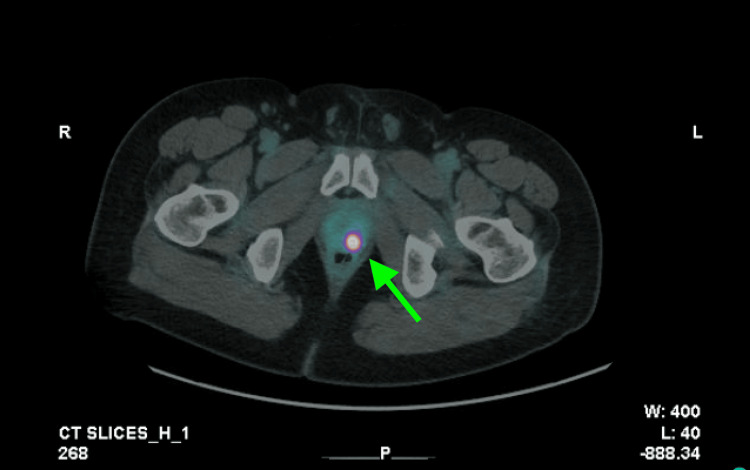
PSMA PET/CT Demonstrating Focal Uptake in the Left Posterior Prostate Axial fused gallium-68 (68Ga) prostate-specific membrane antigen (PSMA) positron emission tomography/computed tomography (PET/CT) image demonstrating focal radiotracer uptake in the left posterior prostate (arrow), corresponding to a suspicious lesion concerning for malignancy.
